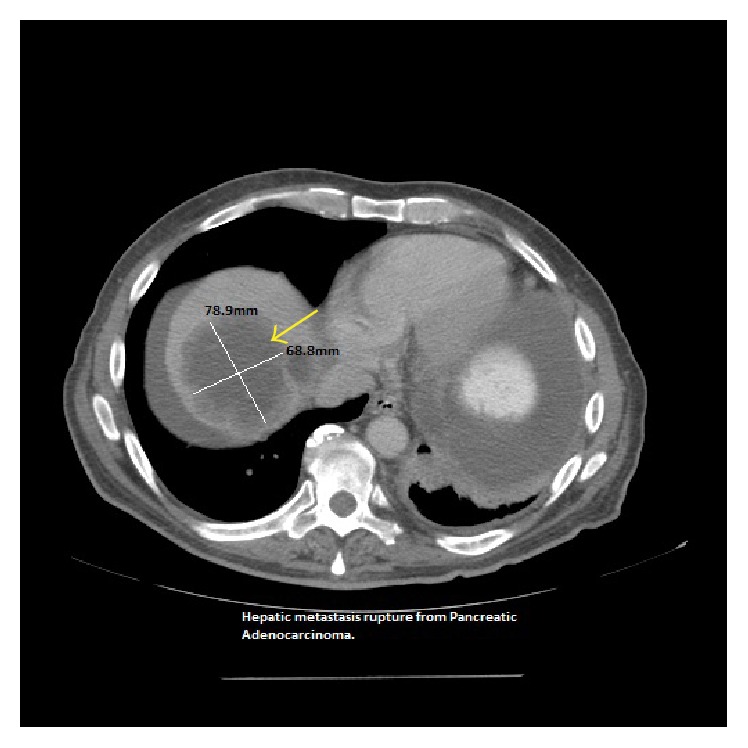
Axial contrast enhanced CT image showing metastasis in liver dome (large yellow arrow) had grown since earlier study (Figure 2).
